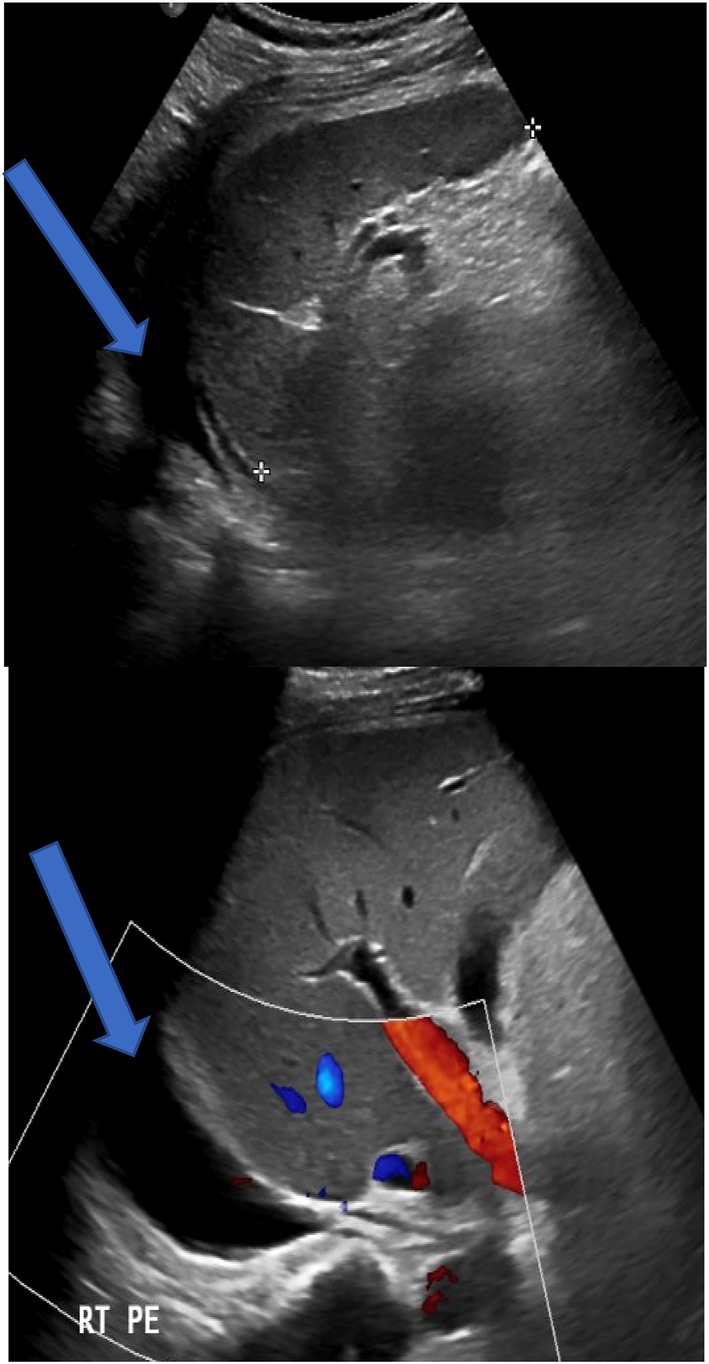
Doppler of liver: Fatty liver; patent hepatic vasculature; mild residual ascites (blue arrows).
